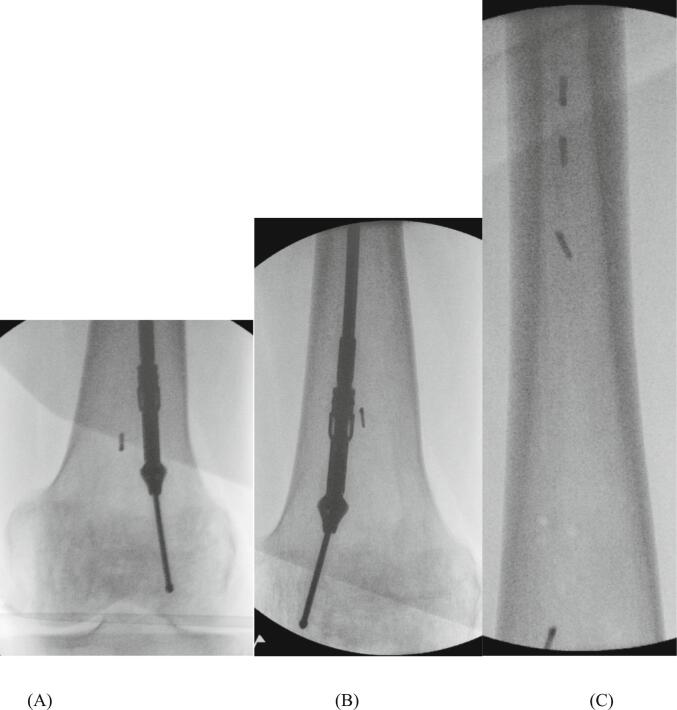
Intraoperative radiographs of small metal debris parts of the broken reamer head in the supracondylar region (A, B), and in the intramedullary canal (C).
